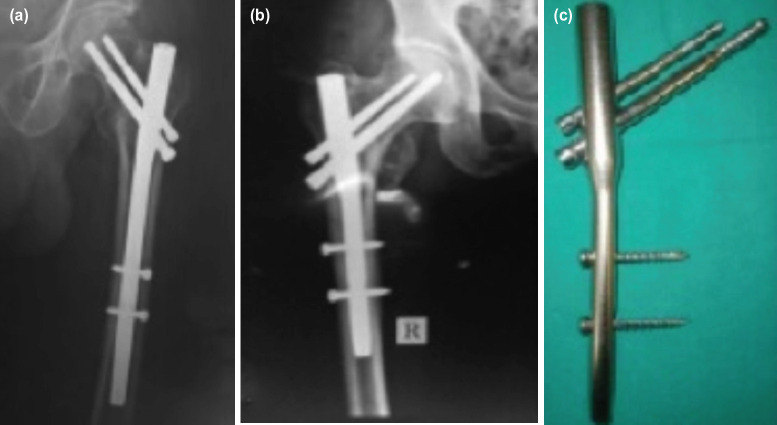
(a) radiograph of standard PFN, (b) radiograph of modified short PFN, (c) modified short PFN : compare how the standard PFN (250mm-on the left) crosses isthmus while modified short PFN stays well short of it.
